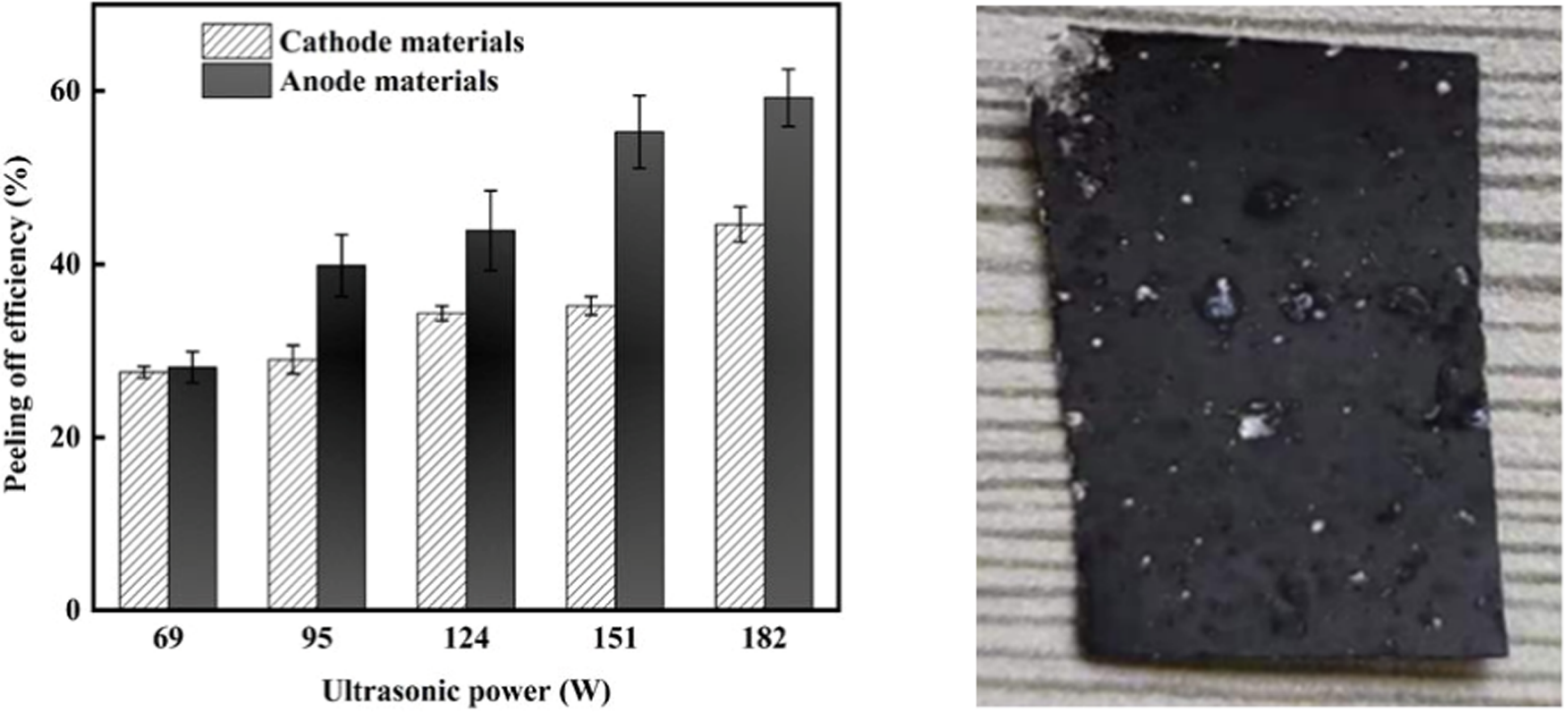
Effects of various ultrasonic powers on
